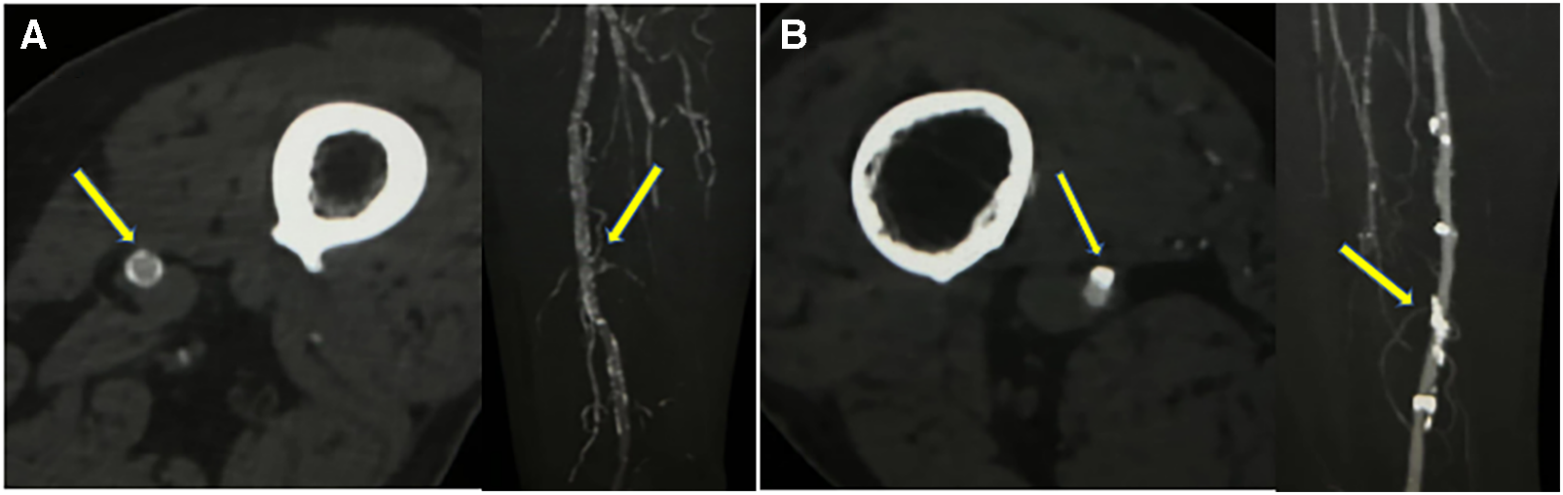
Two different superficial femoral artery calcification patterns on CTA (window width 1,300 Hounsfield Units; window level 300 Hounsfield Units) (A) axial CTA images showed calcifications <1.5 mm, >270° of artery circumference, maximal intensity projection showed continuous calcification (indicating dominant medial calcifications). (B) axial CTA images showed calcifications >1.5 mm, <90° of artery circumference, maximal intensity projection showed continuous irregular calcification (indicating dominant intimal calcifications).
